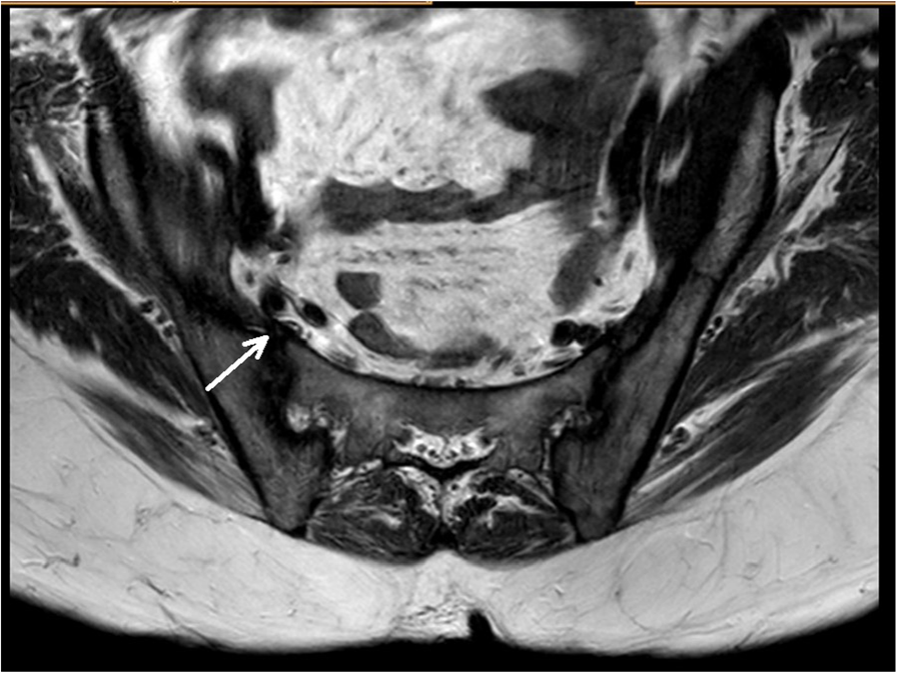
Coronal view of MRI sacroiliac joints: (following completion of intensive phase treatment) There is reduction of oedema over right internal iliacus muscle, iliac bone and sacrum with normal right sacroiliac joint(white arrow)
